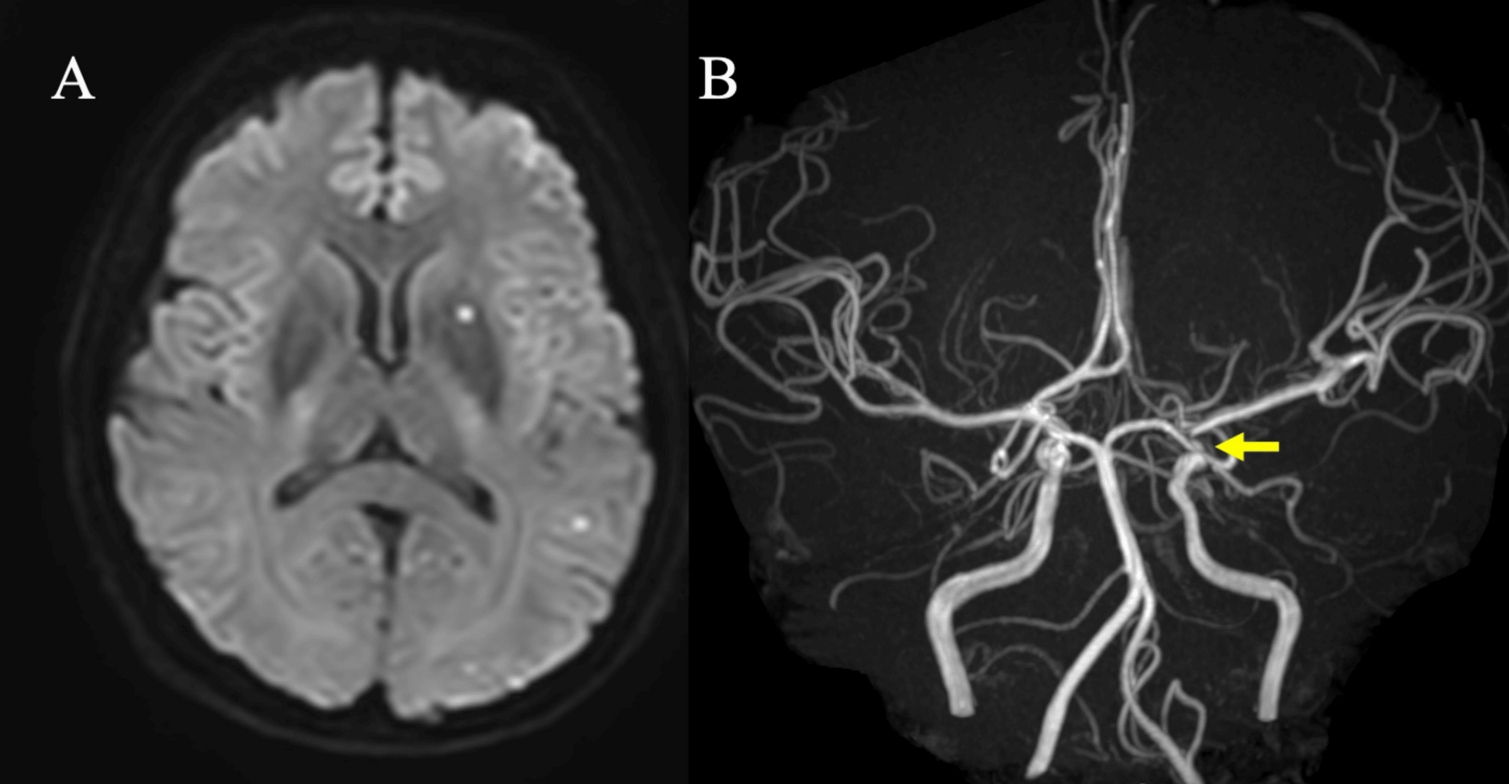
Post-operative MRI images The MRI performed three days post-treatment revealed only several hyperintensity spots on the DWI (A). There were no findings indicative of vessel occlusion due to stent placement, and a left-paraclinoid aneurysm was not identified on the magnetic resonance angiography (B, yellow arrow).
